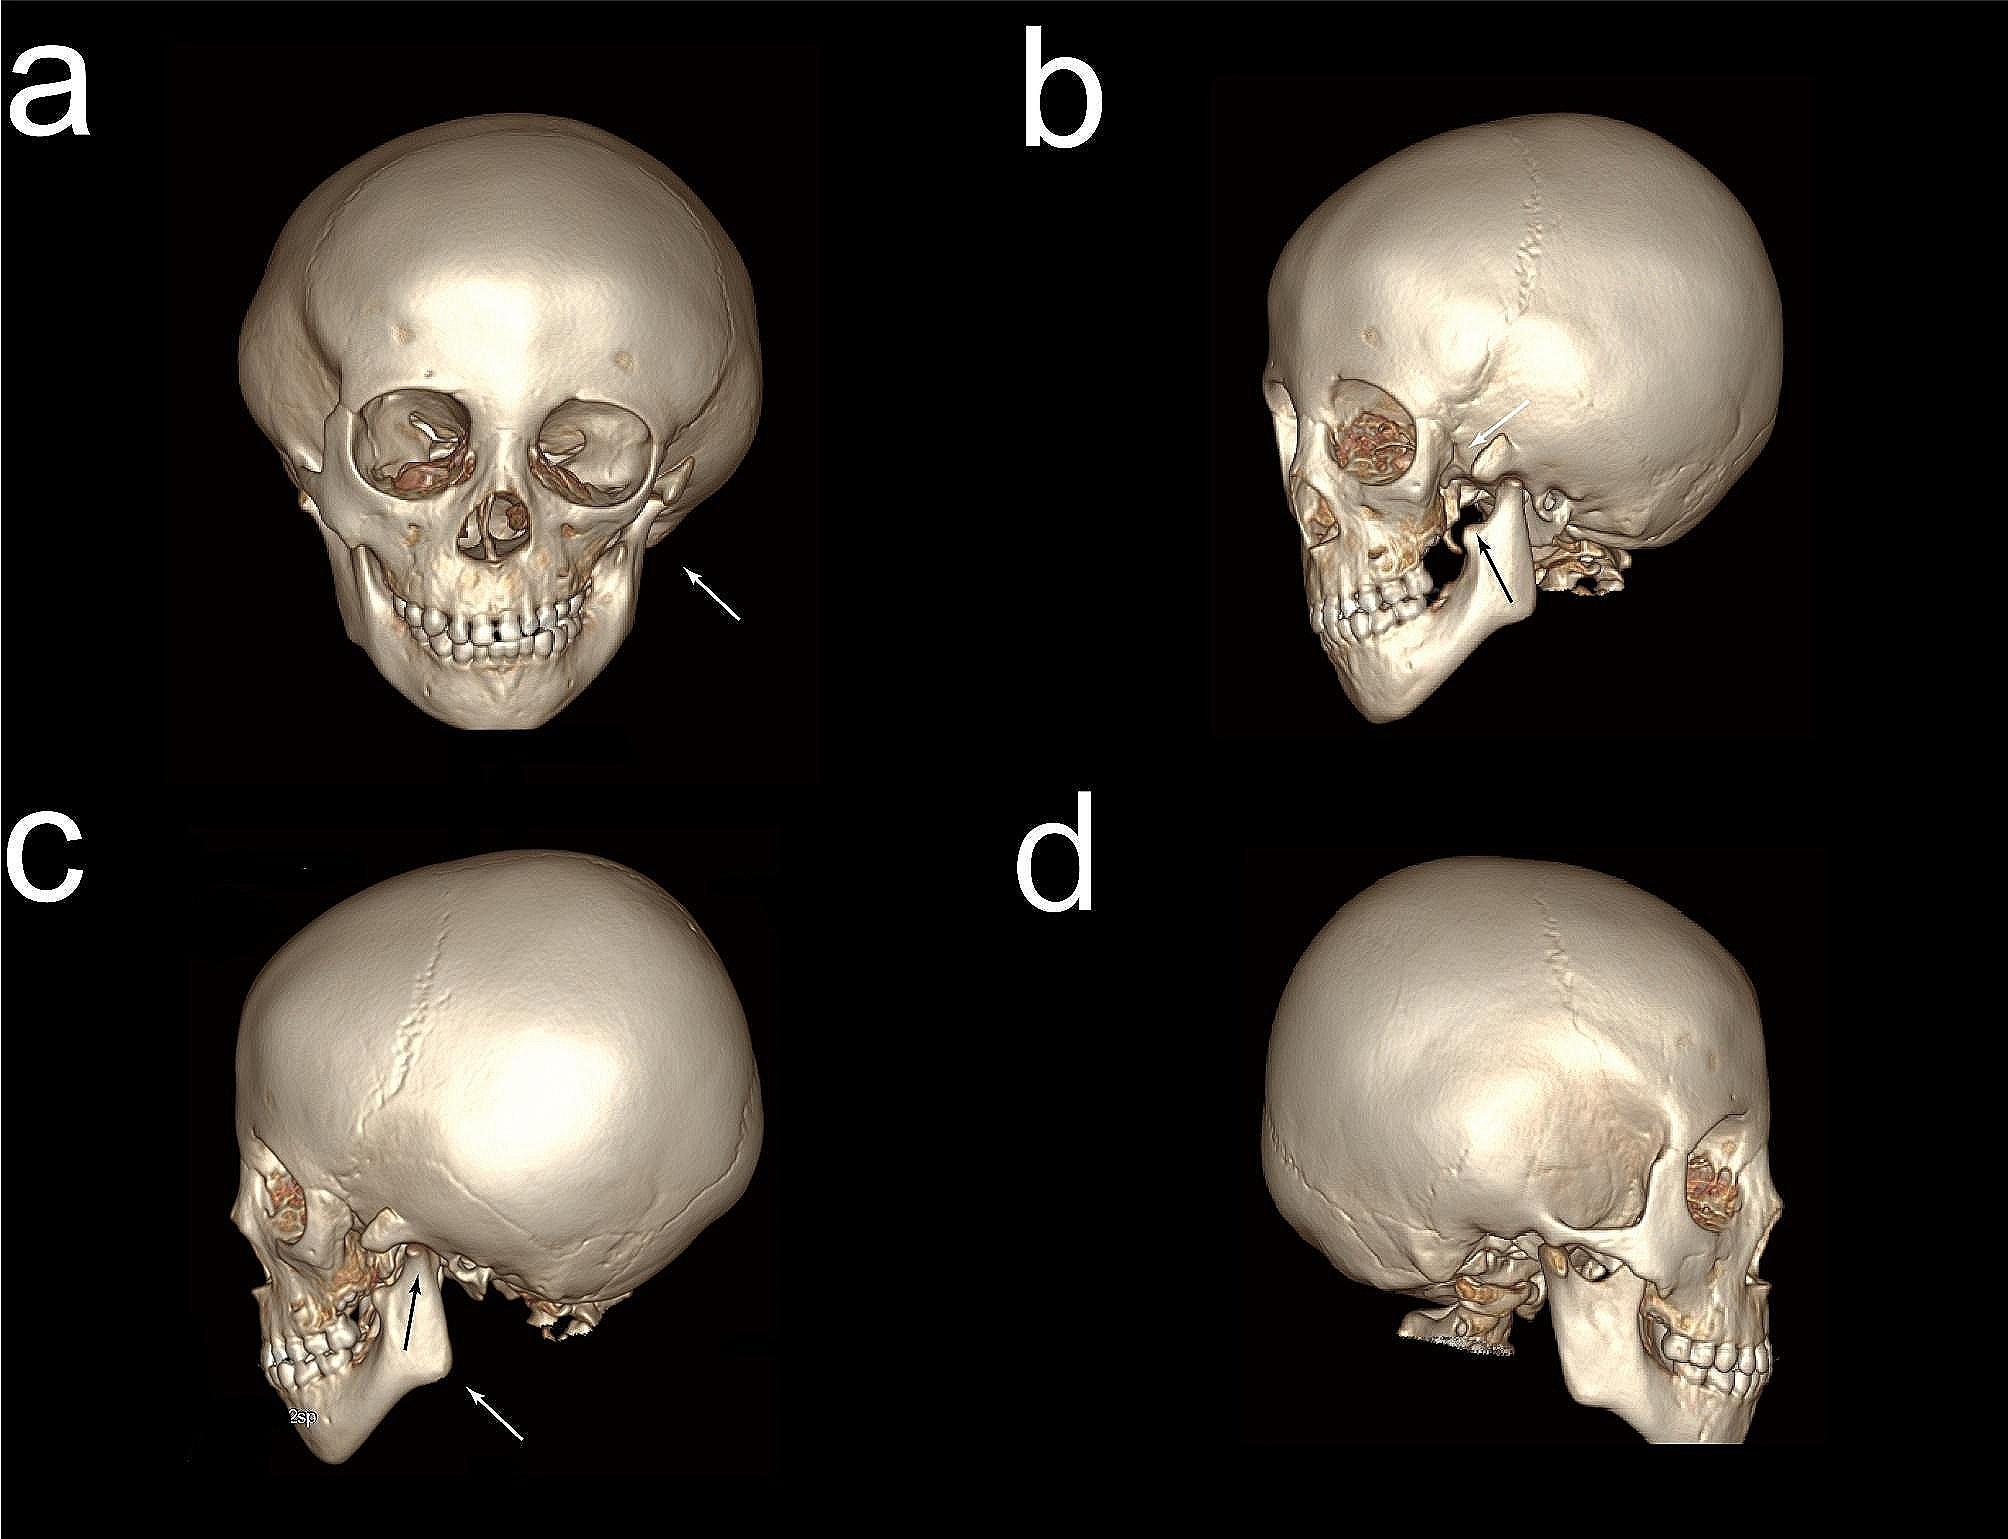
The skull CT scan of the patient. (a) Skull CT scan (white arrow) showed asymmetry of the bilateral mandible and zygomatic bone. (b) The left zygomatic bone lacks a temporal process (white arrow). And there is agenesis of the coronoid process of the mandible (black arrow). (c) Hypoplasia of the mandibular (white arrow) and the condyle (black arrow) on the left side. (d) CT scan of normal skull on the right side
